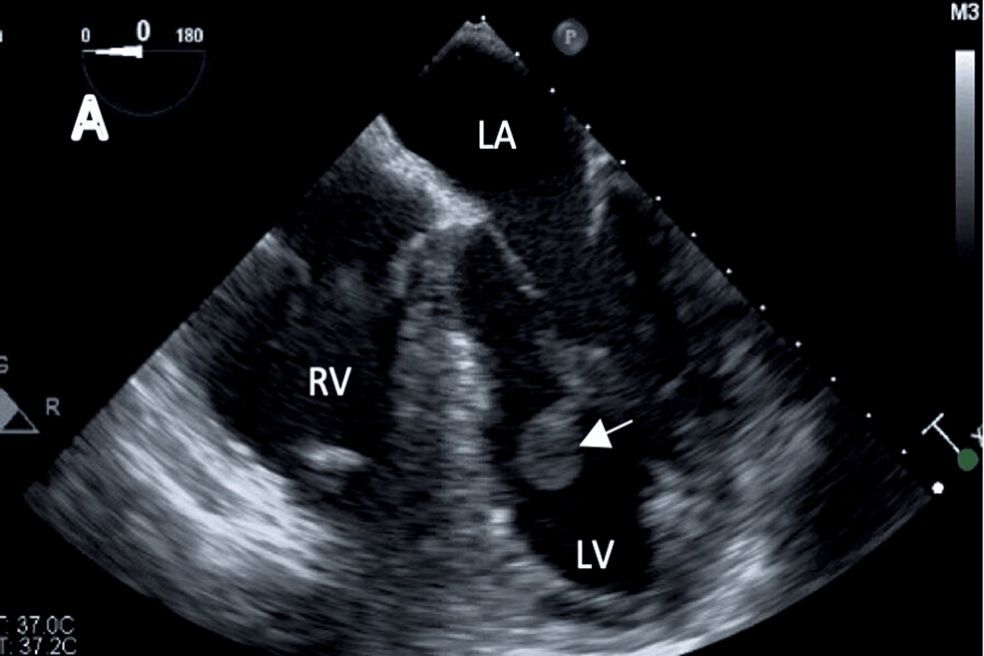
Transesophageal echocardiogram TEE images from various mid-esophageal angles, positioned to reveal a large pedunculated mass (white arrow) in the left ventricle (LV), attached to the chordae of the papillary muscle of the mitral valve LA: left atrium; RV: right ventricle
